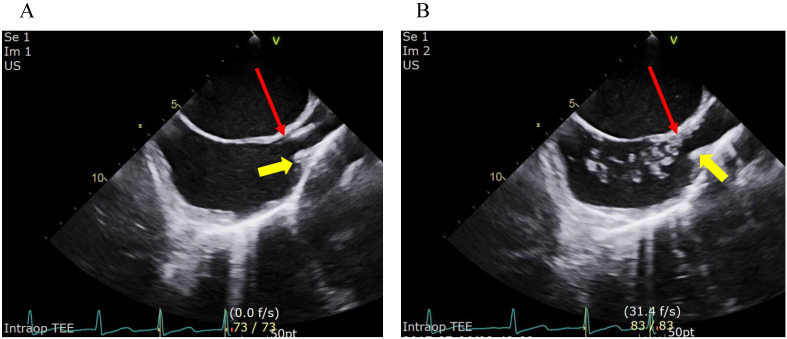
Confirmation of the placement of the catheter tip at the superior edge of the crista terminalis on the bicaval view of transoesophageal echocardiography. Figure 1A depicts placement of the catheter tip at the crista terminalis on the bicaval view of transoesophageal echocardiography. Figure 1B depicts the use of microbubbles to check the location of the catheter tip when the position of the tip was vague. Multiple hyperechogenic microbubbles were seen in the right atrium when the normal saline was rapidly injected through the port of the distal lumen of the central catheter. The yellow arrows indicate the superior edge of the crista terminalis. The red arrows indicate the catheter tip.
